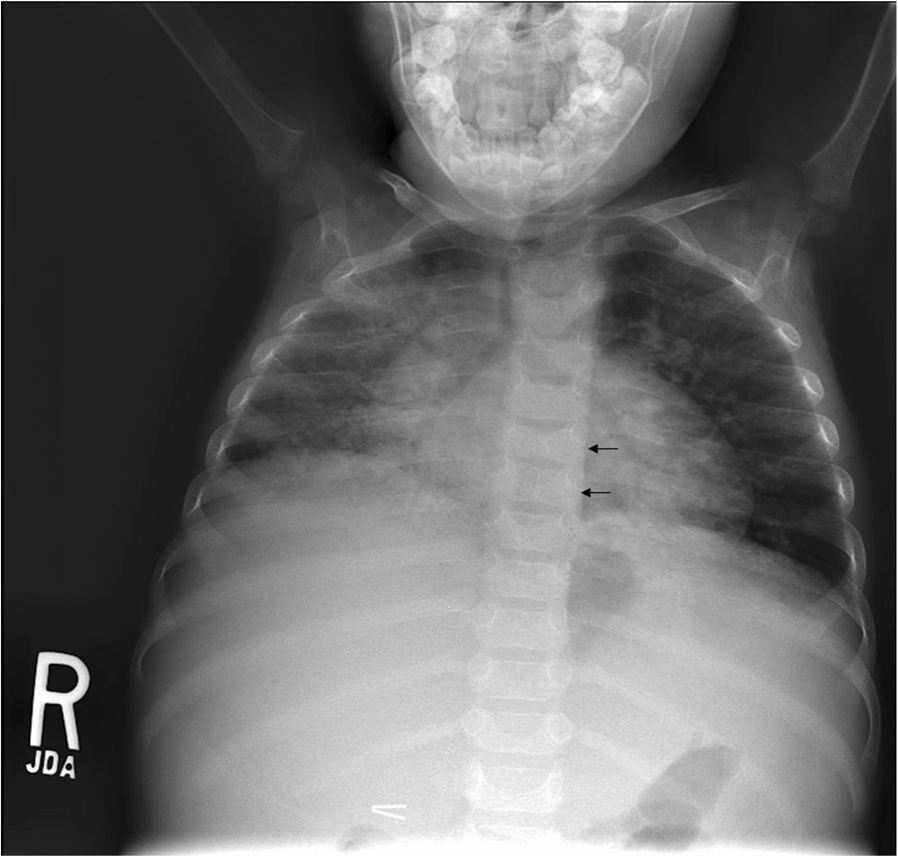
Chest radiograph consistent with Alagille syndrome and sarcoidosis. Anterior view chest radiograph revealing extensive bilateral chronic lung disease, mediastinal adenopathy, butterfly vertebrae (arrows), and hepatomegaly.
